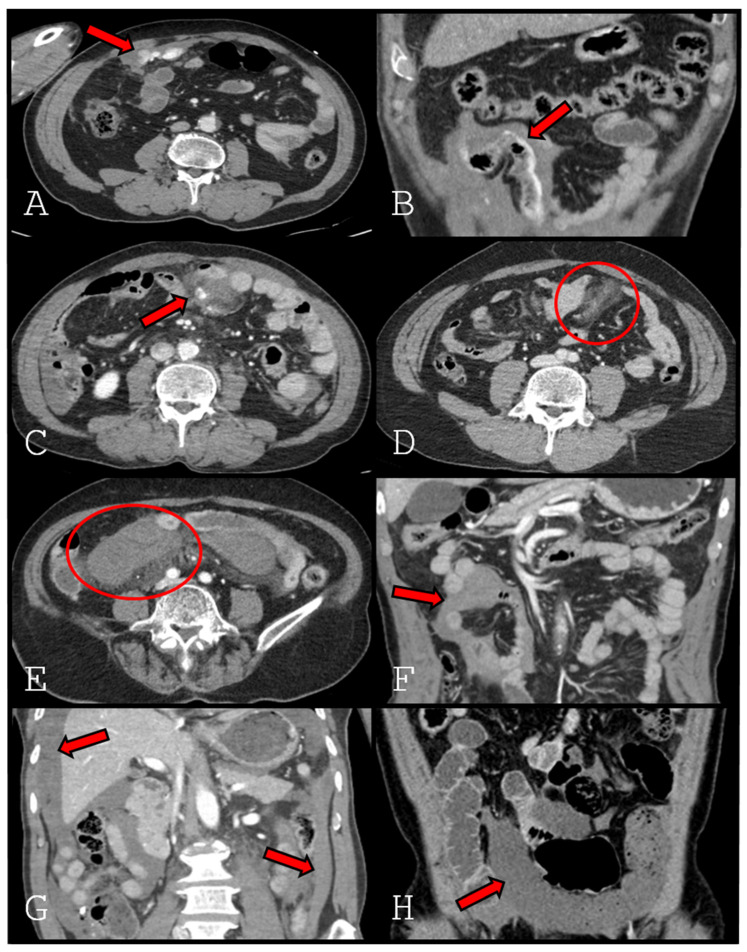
Representative CT findings in mesenteric injury after blunt trauma. (A). Extravasation type 1 (arrow). (B). Extravasation type 2 (arrow). (C). Pseudoaneurysm (arrow). (D). Mesenteric haziness (circle). (E). Mesenteric hematoma (circle). (F). Interloop fluid (arrow). (G). Dependent portion fluid (arrow). (H). Decreased bowel wall enhancement (arrow).
